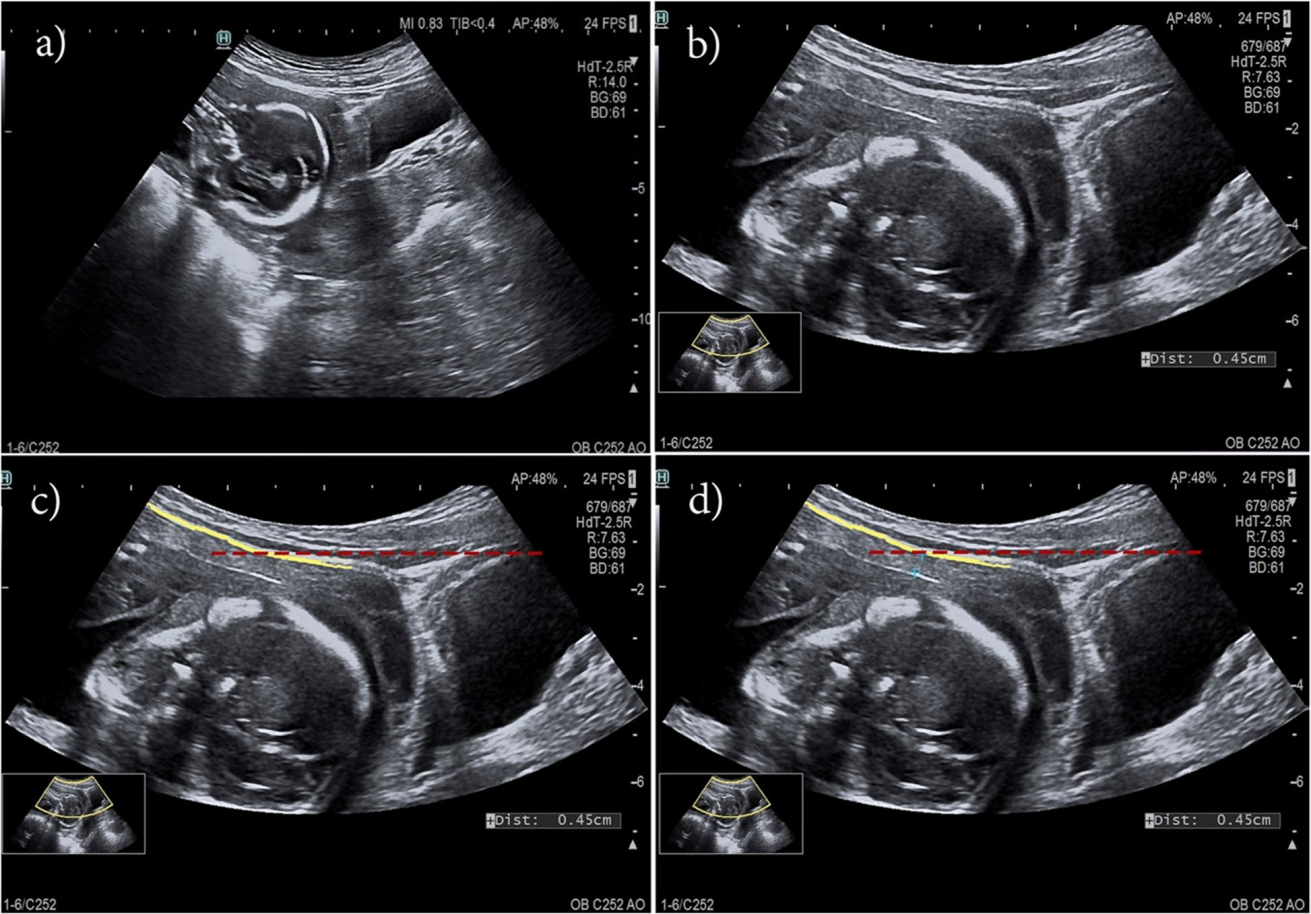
The measurement of the LUW thickness. a An ultrasound transducer was placed in sagittal plane just above the pubic symphysis to visualize the urinary bladder, cervix with visible cervical canal, and LUW. b The picture was enlarged until LUW and the urinary bladder occupied the majority of the screen. c A virtual red dashed line was drawn in horizontal plane between the dome of urinary bladder and LUW to mark a point for measurement. The yellow line represented the peritoneum that covered the LUW. d The calipers were placed in inner-to-inner fashion to measure the LUW thickness
